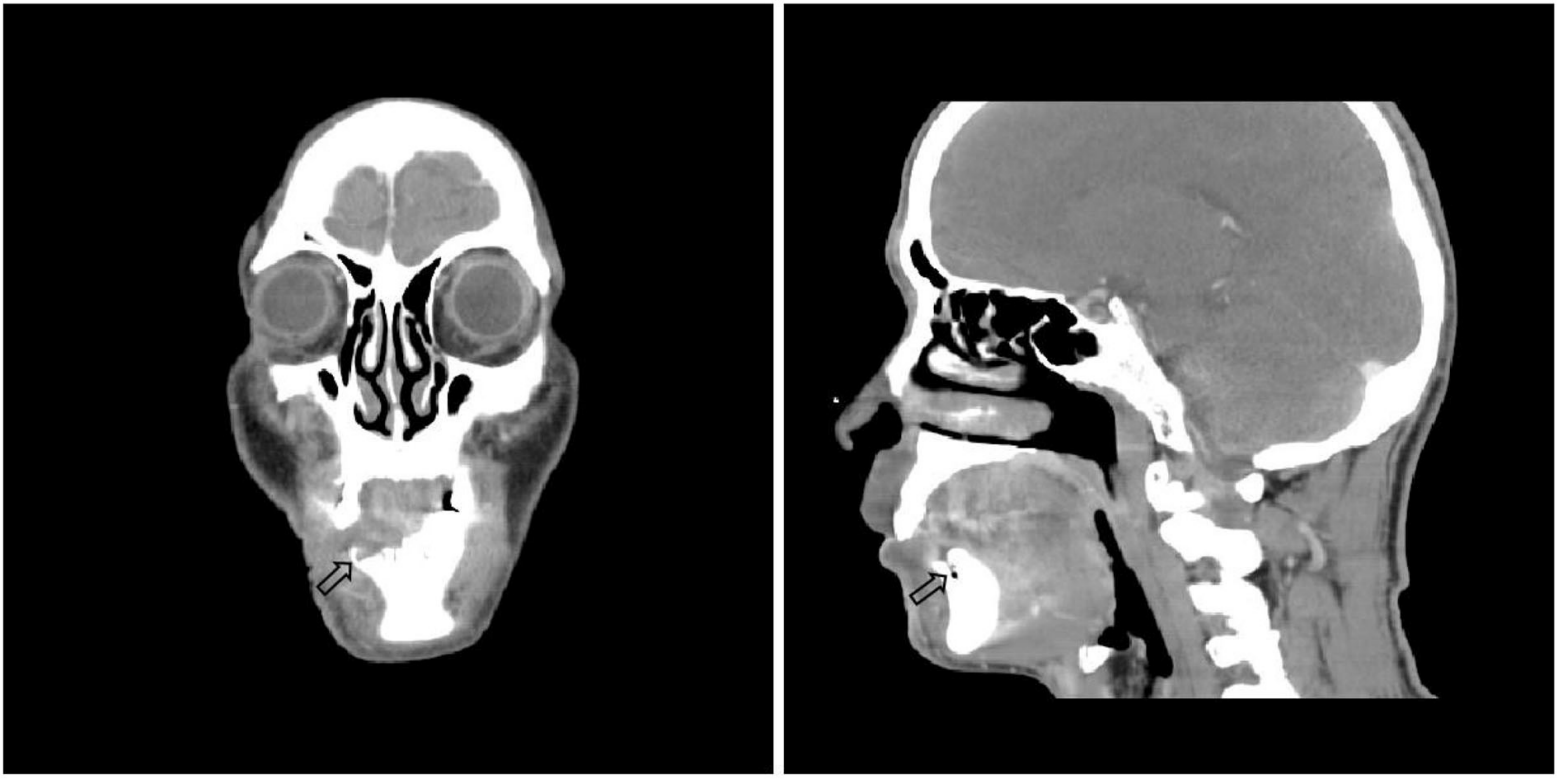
Cranial neck enhanced CT(2021-4-30) showed the right side of the lower alveolar bone is partially absorbed, and there is no corresponding tooth. No tumor or other suspicious lesions.
